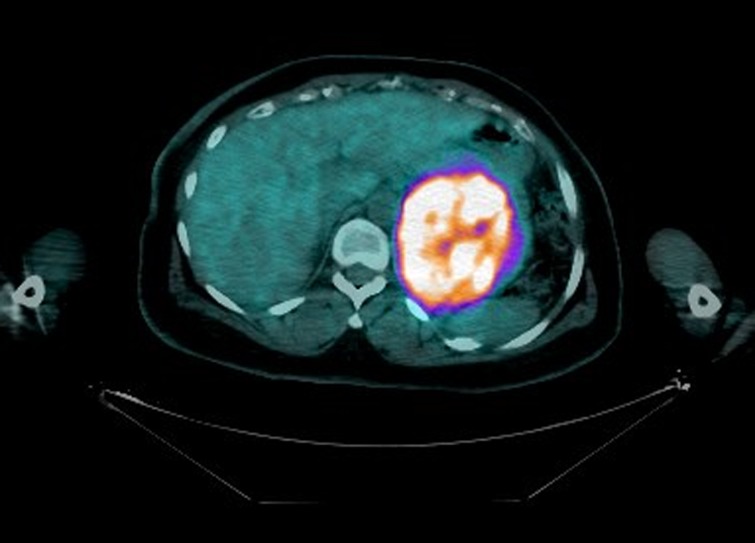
^18^FDG PET showing increased uptake in the left adrenal mass with SUVmax of 25.
